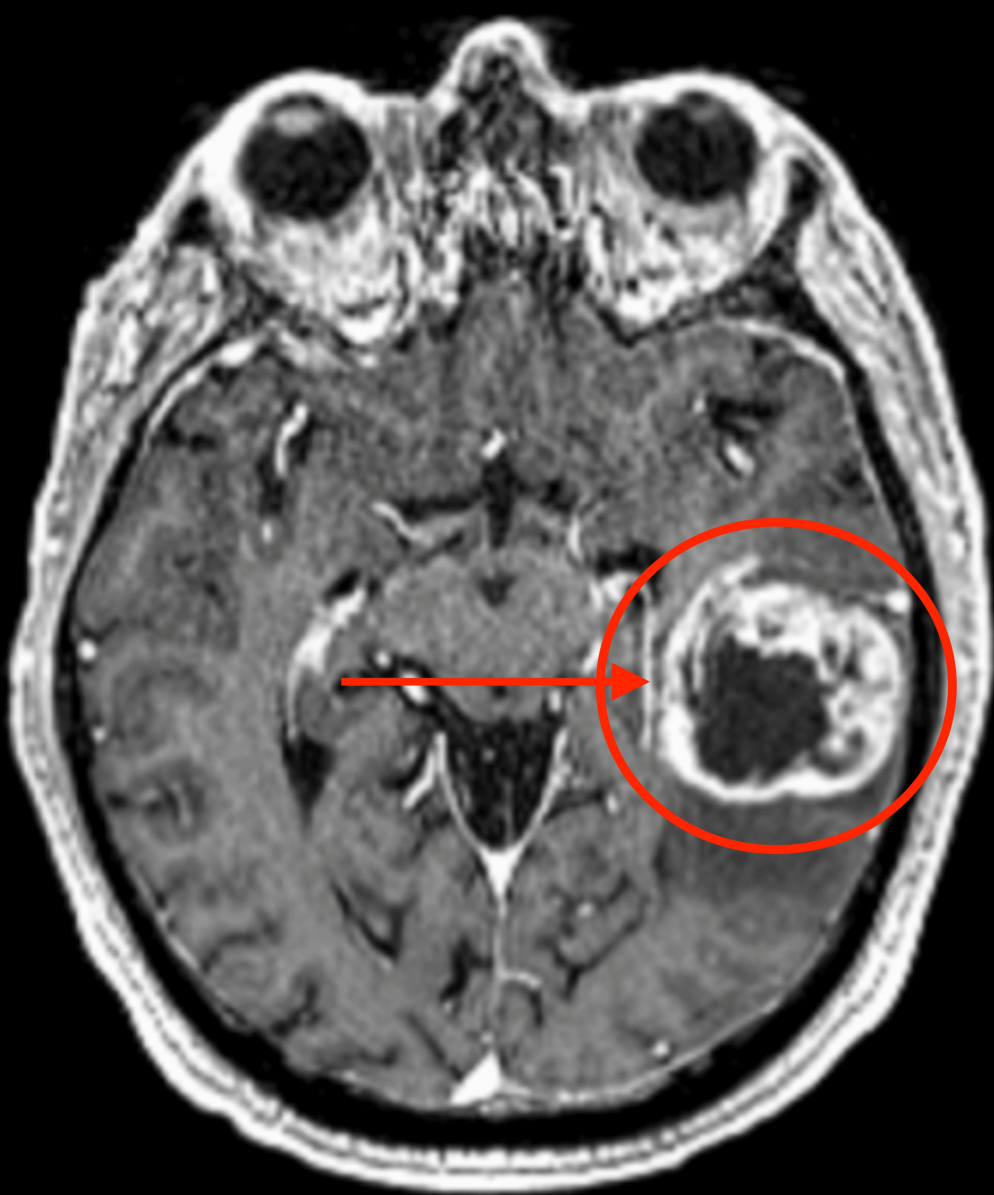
Axial T1-weighted MRIs after IV gadolinium administration The figure depicts glioblastoma and how it looks under a traditional MRI after administering gadolinium contrast using IV. Image Source: American Association of Neurological Surgeons: Glioblastoma multiforme [2]; Used with permission from the American Association of Neurological Surgeons.
